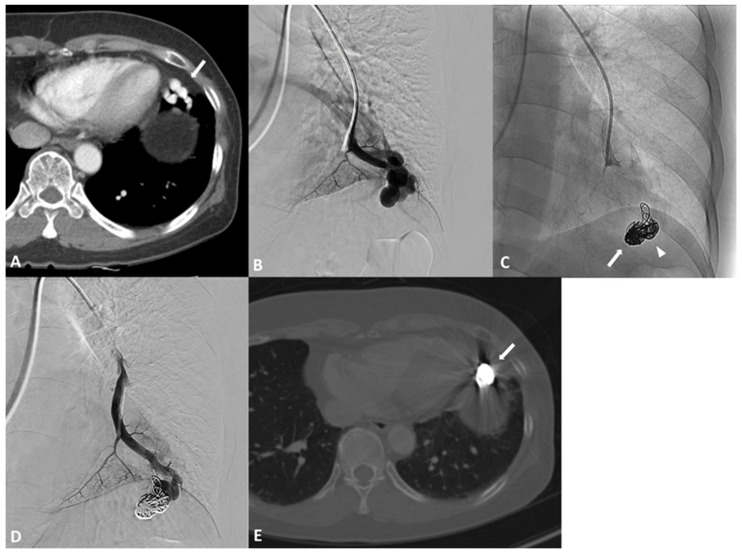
A 62-year-old woman with pulmonary arteriovenous malformation (PAVM). (A) Contrast-enhanced chest CT shows a PAVM in the left lower lobe (arrow). (B) Selective pulmonary angiography of the feeding artery confirms the lesion. (C) A 10 mm 3D coil is placed in the venous sac as the initial framing coil (arrow), followed by additional coils (arrowhead). (D) Final angiogram demonstrates complete occlusion of the venous sac, with no visible draining vein. (E) Follow-up CT at 3 months shows densely packed coils within the PAVM (arrow) and regression of the draining vein.
